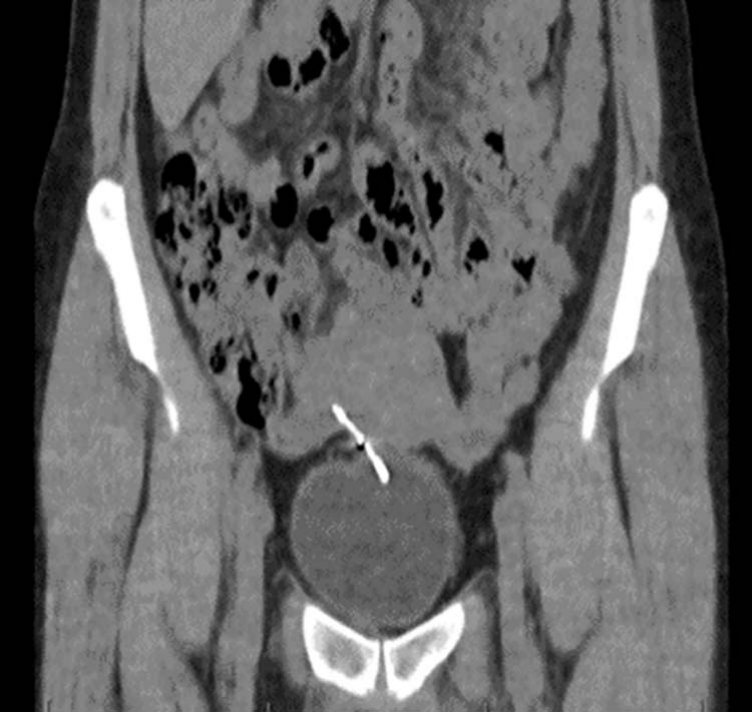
Coronal CT urinary tract image demonstrating the device lying between uterus and bladder, with one horn of the device embedded into the bladder.
